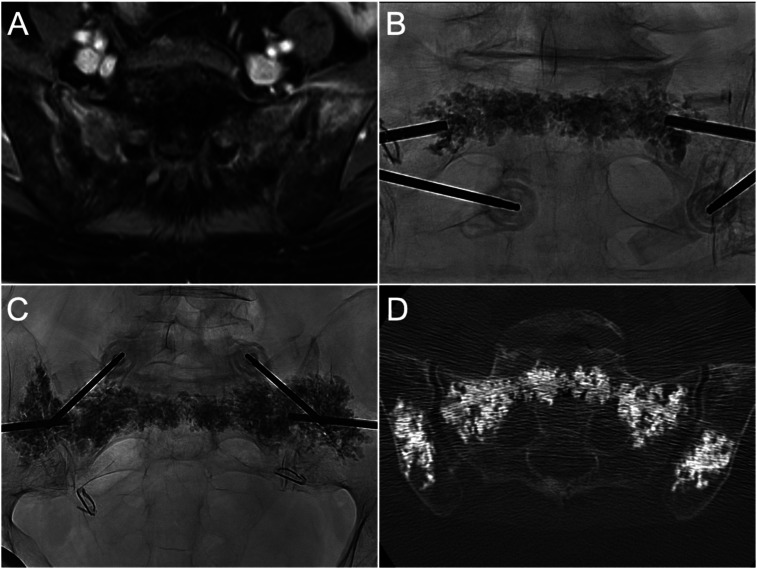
Co-axial sacroplasty and iliac cementoplasty for bilateral sacral and iliac insufficiency fractures, in a patient previously treated with pelvic radiotherapy for cervical cancer. (A) Axial T1FS with gadolinium demonstrates contrast enhancement in the sacral ala and iliac bones consistent with insufficiency fractures. (B) PA radiograph demonstrates cement distribution in the sacral body (Zone I) and periforaminal regions (Zone II) after sequential injections. (C) PA radiograph shows contiguous cement distribution from ala-to-ala at S1 following sequential cement injections, and bilateral iliac cementoplasties. (D) Axial CT image shows cement overall distribution and absence of cement leakage.
